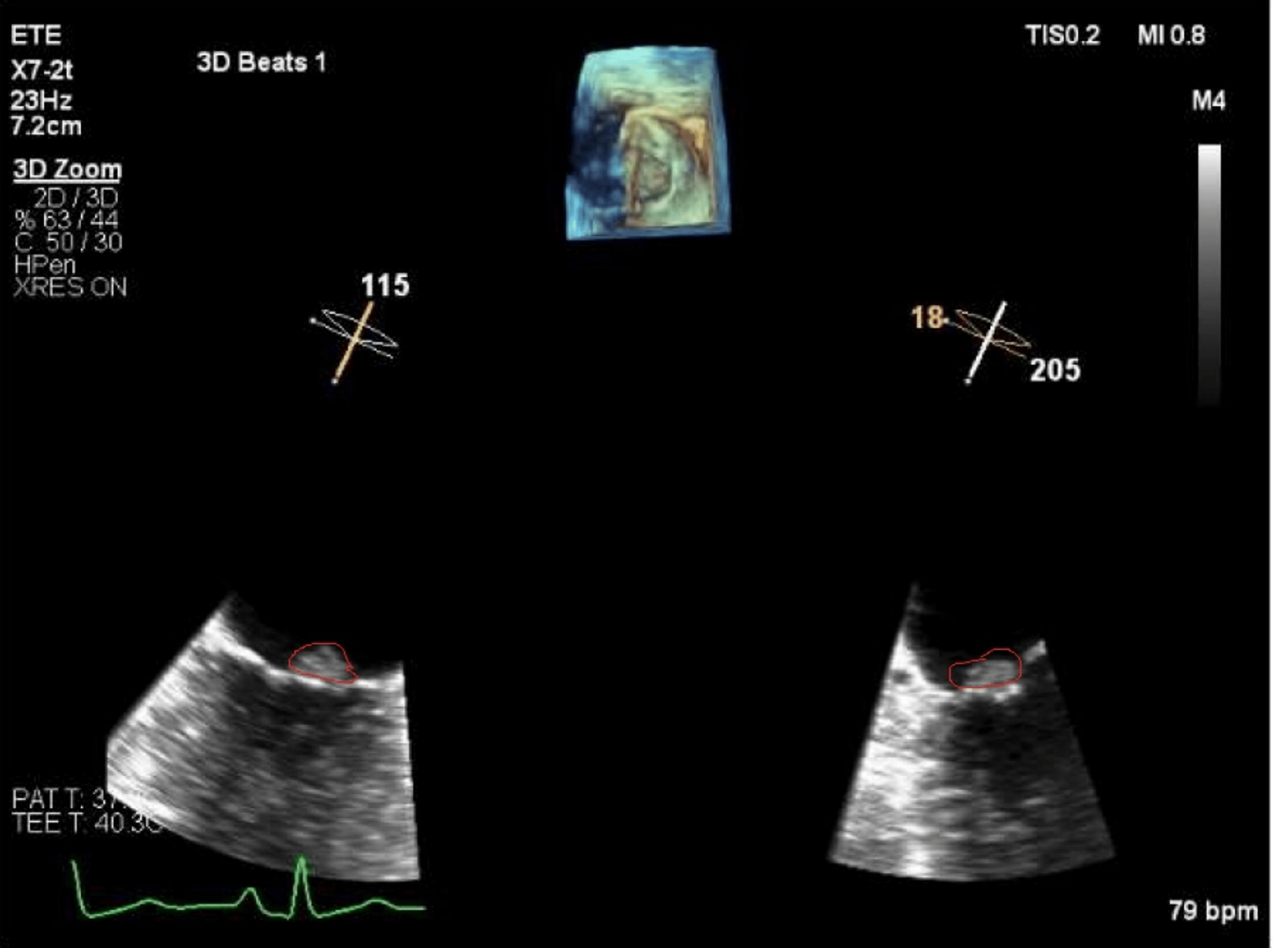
Three-dimensional transesophageal echocardiography (3D TEE) Image showing a rounded lesion attached to the atrial side of the posterior leaflet of the mitral valve.
